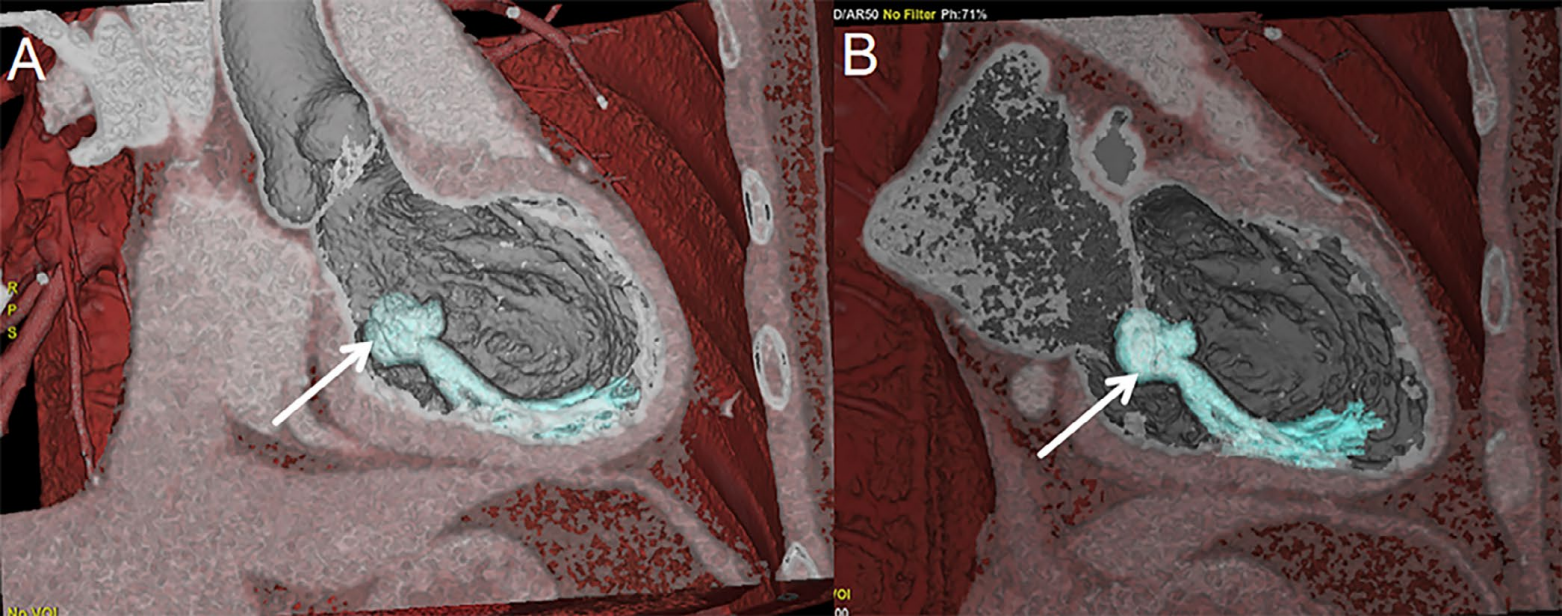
The features of case 1 on cardiac CT. Through cardiac CT (A, B), it can be seen that circular tissue resembling myxomas and fibroids attached to the mitral valve, as well as tendon‐like structures connected to this tissue, is attached to the ventricular wall (shown by the white arrow in the figure).
